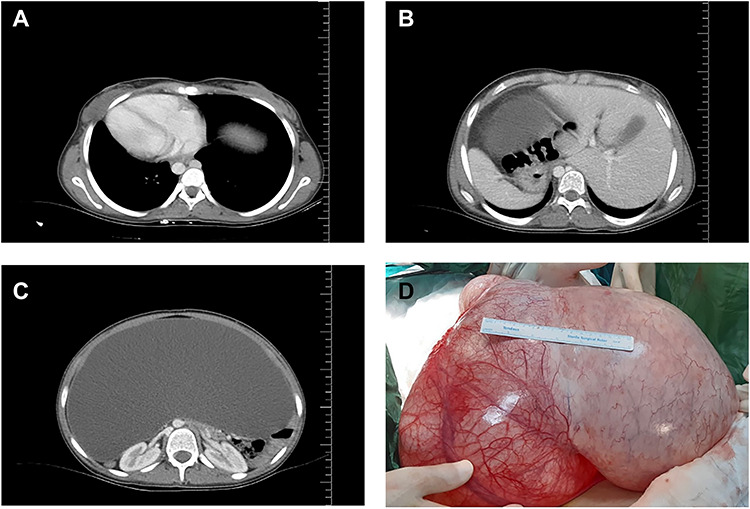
Organ locations (A, B) showing situs inversus totalis and axial (C) and coronal (D) views of the cystic mass on computed tomography.
